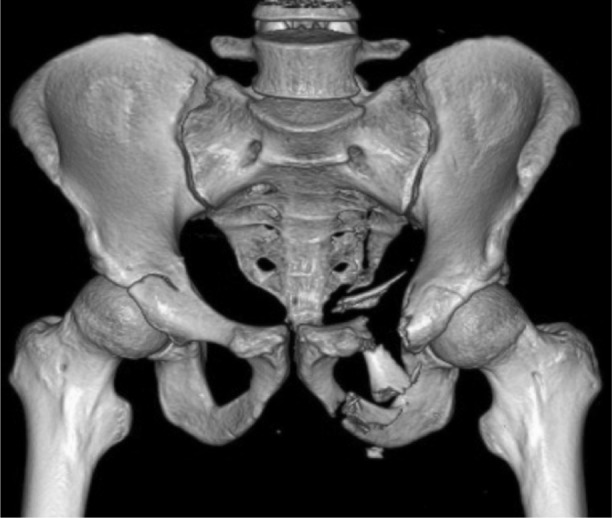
3D Computed Tomography (CT imaging) showing left superior and inferior pubic rami fracture, and opening of left sacroiliac joint.
